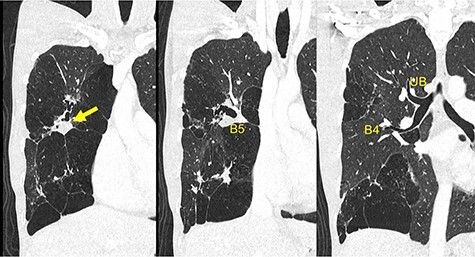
Preoperative chest CT demonstrates a mucinous impaction (Arrow) and blind-end of B5. CT, computed tomography; B, bronchus; UB, upper bronchus.
